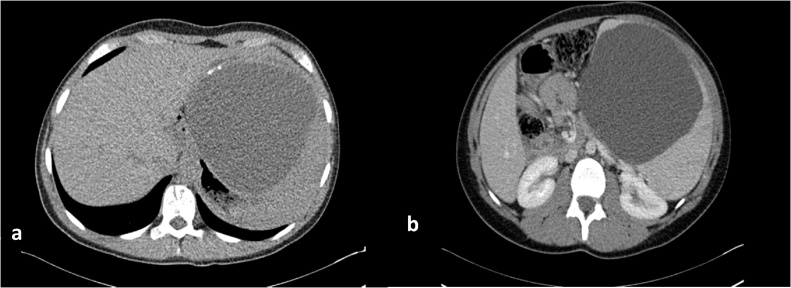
(a) Axial image of non-contrast CT abdomen shows the large splenic cyst with focal peripheral calcification in wall (White arrow), (b) contrast enhanced CT shows no peripheral enhancement, no floating membranes or enhancing solid component.
